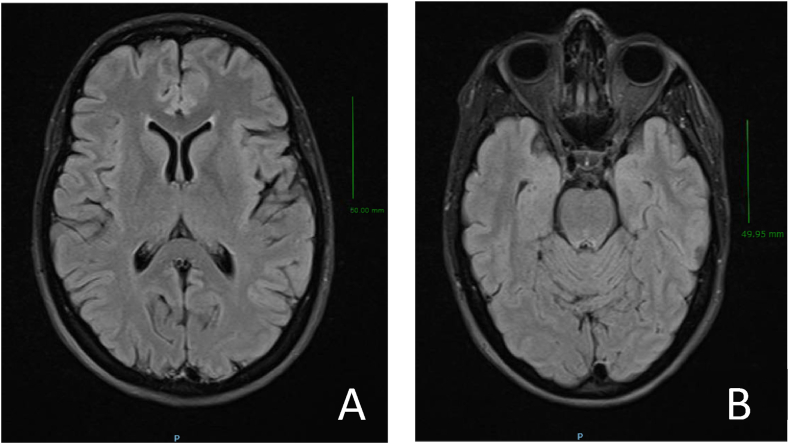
Magnetic resonance imaging (MRI, scale bar 5 cm) of the brain (A) and orbits (B).
